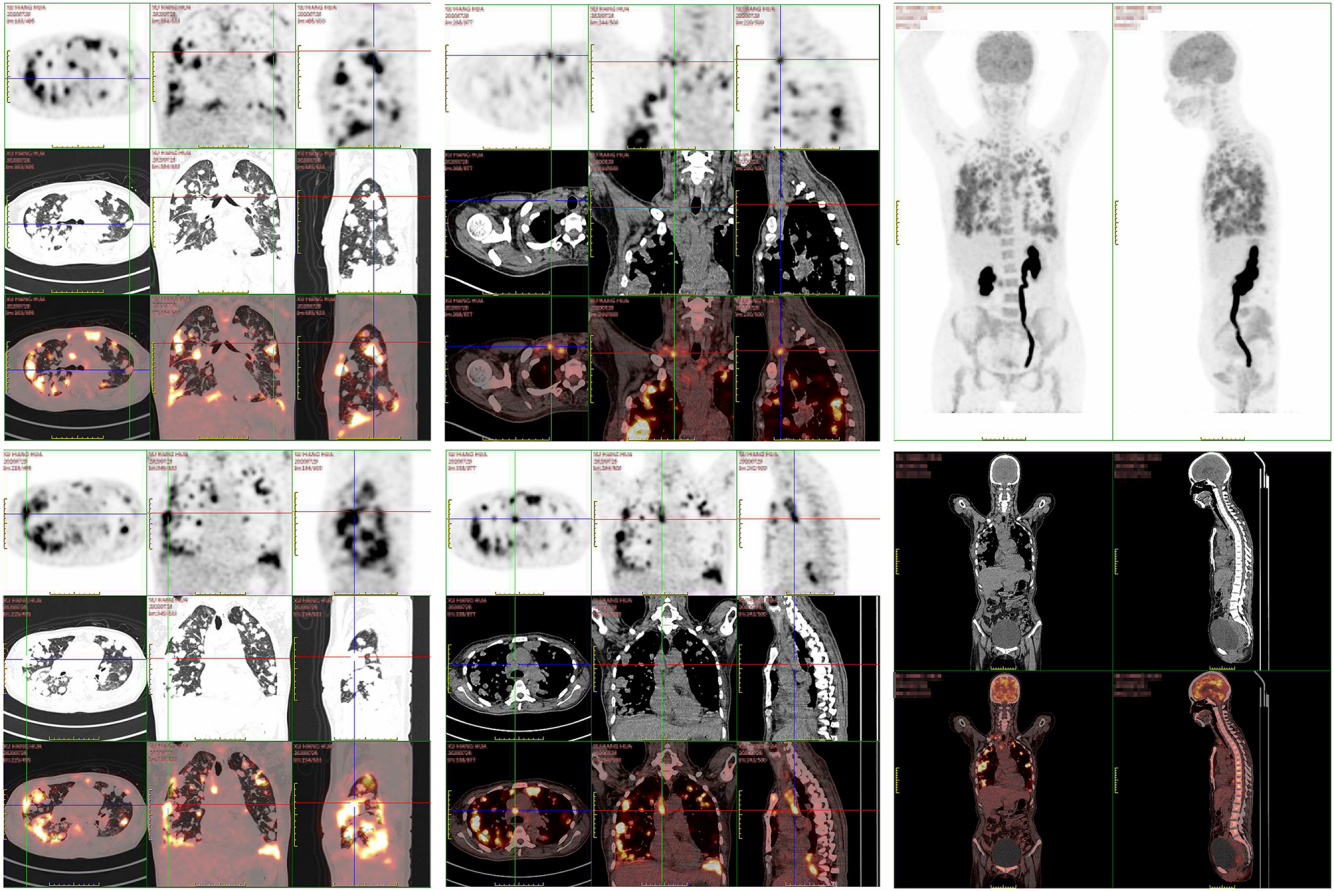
The patient’ s PET/CT scan showed diffused lesions with increased FDG uptake like chest CT located in the lung and LNs without distal metastasis.
